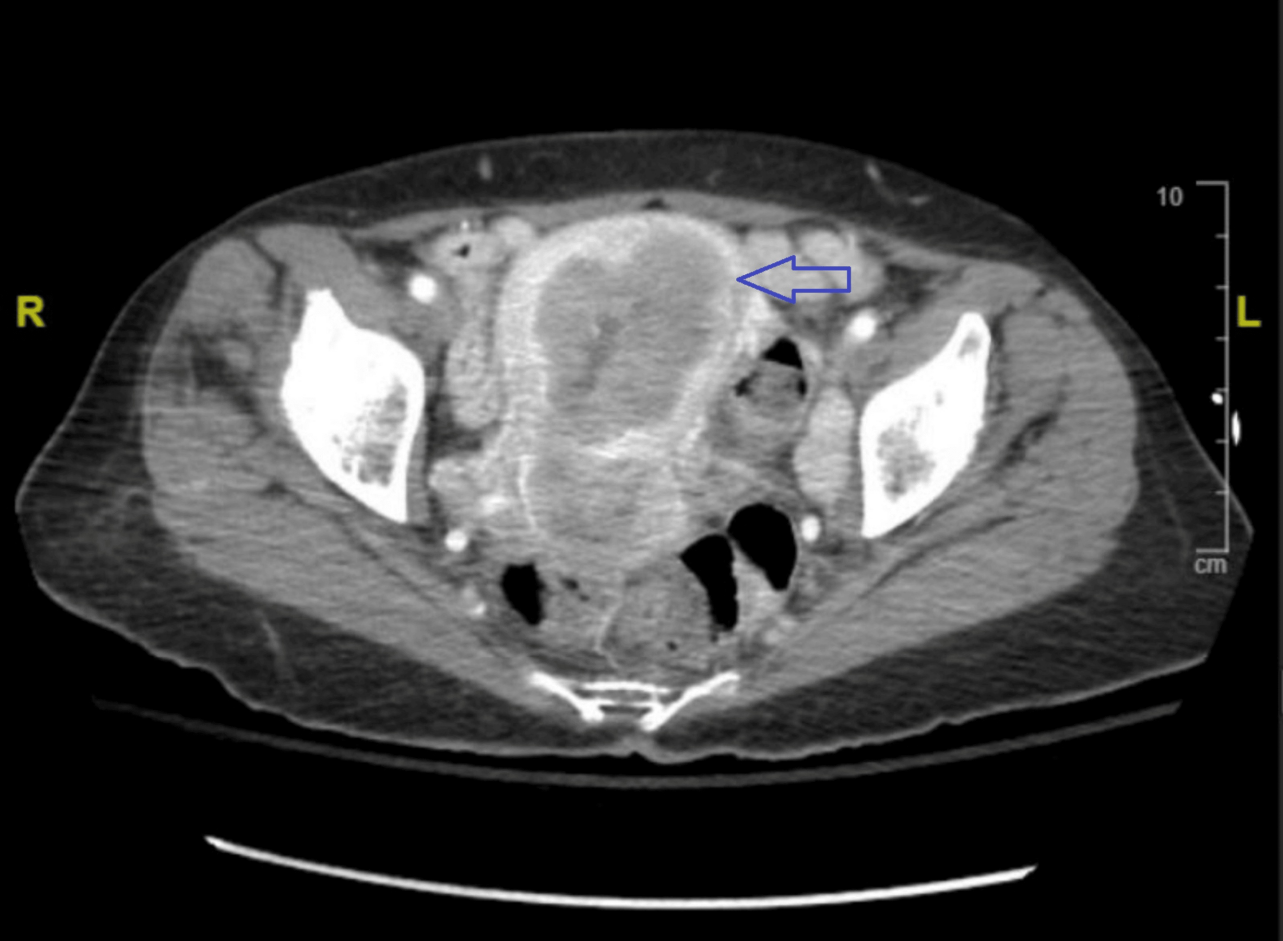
Computed tomography of the abdomen and pelvis demonstrating uterus with endometrial enlargement, the blue arrow points to the uterus
